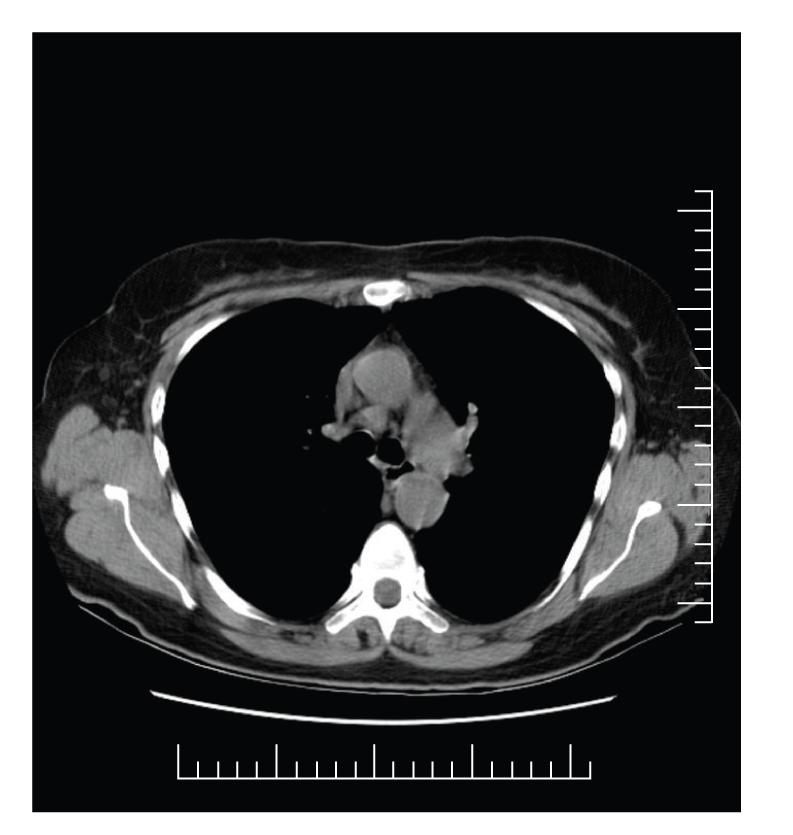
CT Chest showing mediastinal lymphadenopathy.
